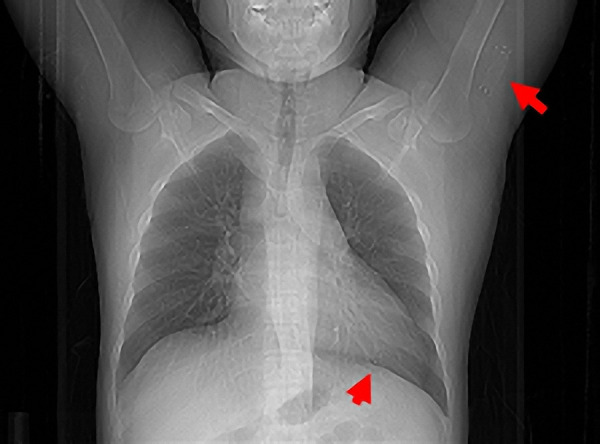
Chest X-ray demonstrating a venous stent in the left upper extremity with 4 heads in distal segment and 3 heads in proximal segment and a small metallic density over the left lung base (red arrows).
